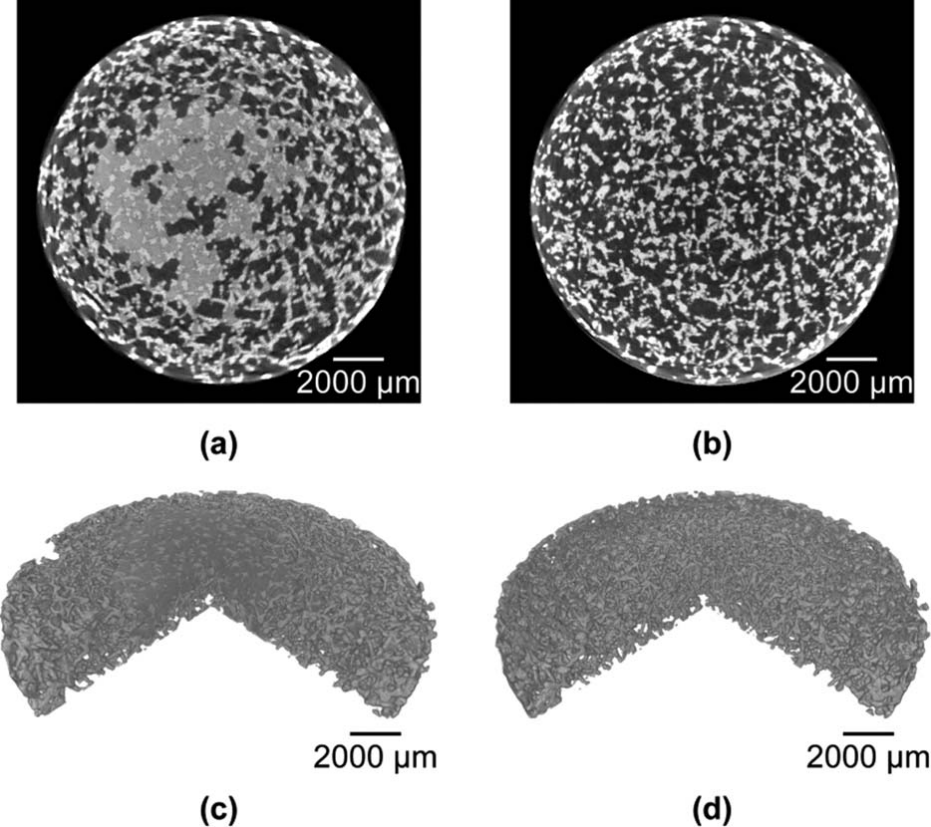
Micro‐CT scans of one of the test cylinders. (a, c) Before acoustic cleaning. (b, d) After acoustic cleaning in the cleaning chamber of the PAS 75 horn.
